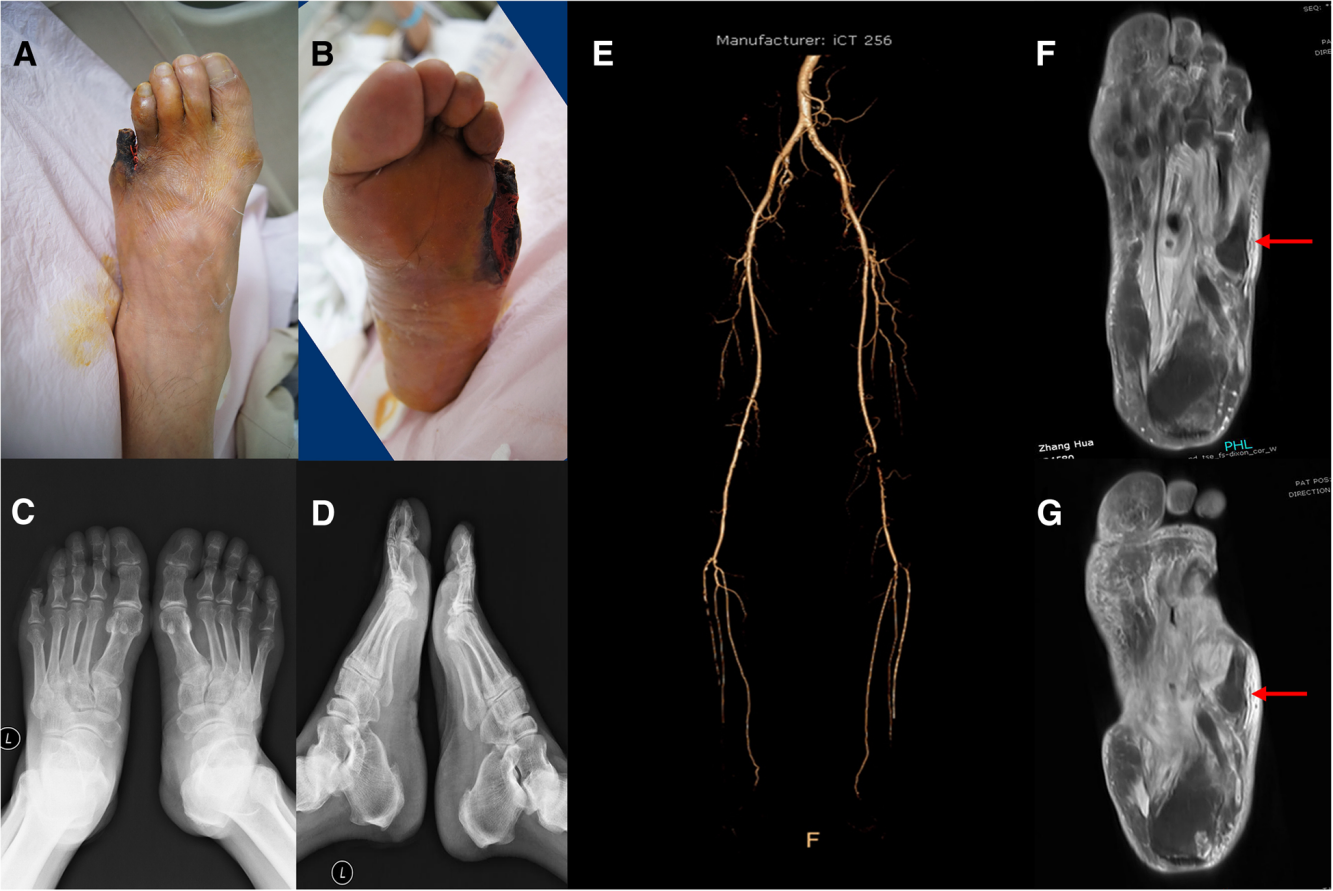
Diagnostic assessment of the diabetic foot ulcer. ab, 10/7/2017; cd, foot X-ray; e, Lower extremity CTA. 13/7/2017. f, foot MRA,14/7/2017. g, foot MRA, 4/9/2017
